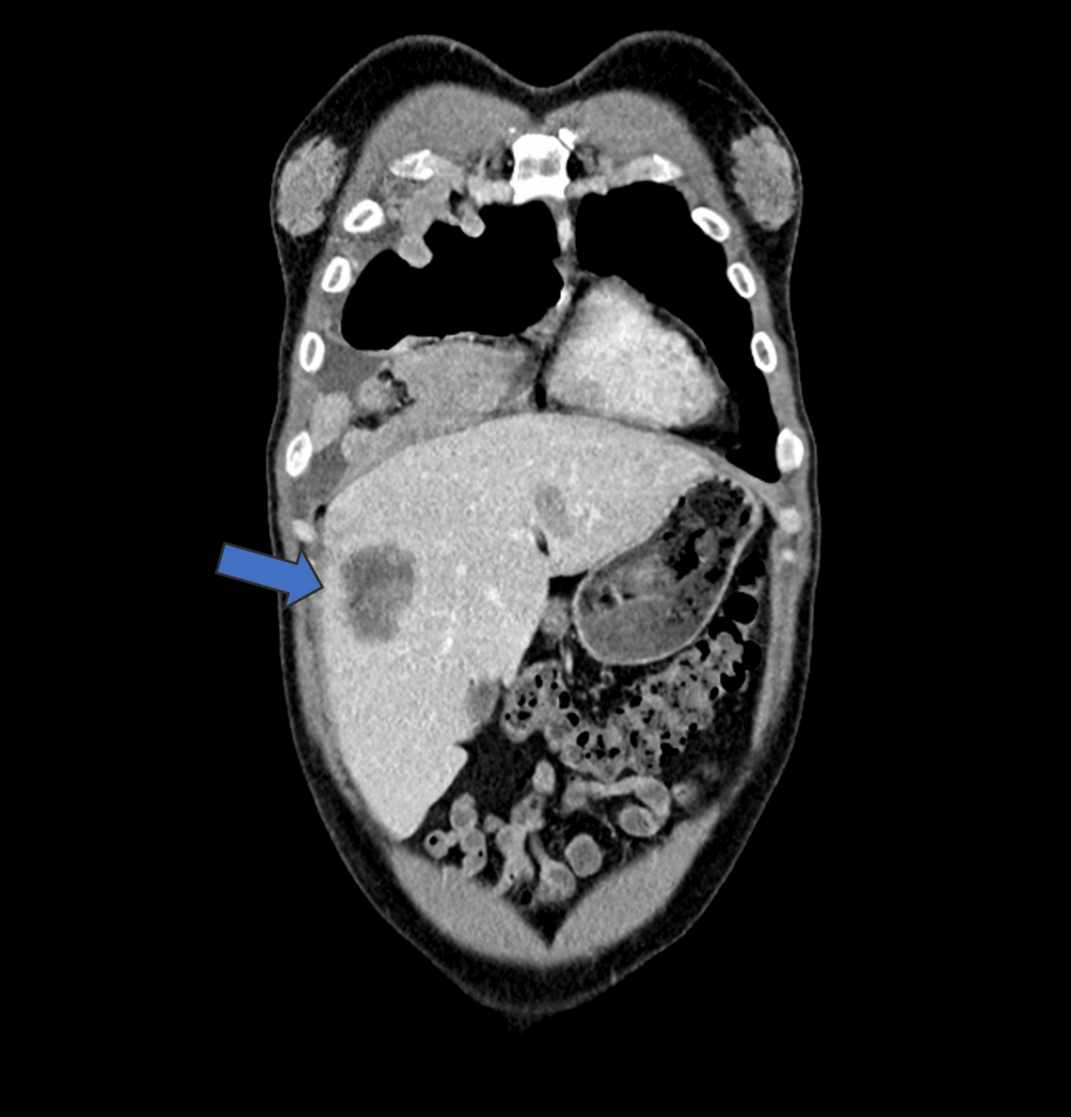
Thoracoabdominopelvic computed tomography (CT) demonstrating a right pleural effusion and hepatic metastases.
